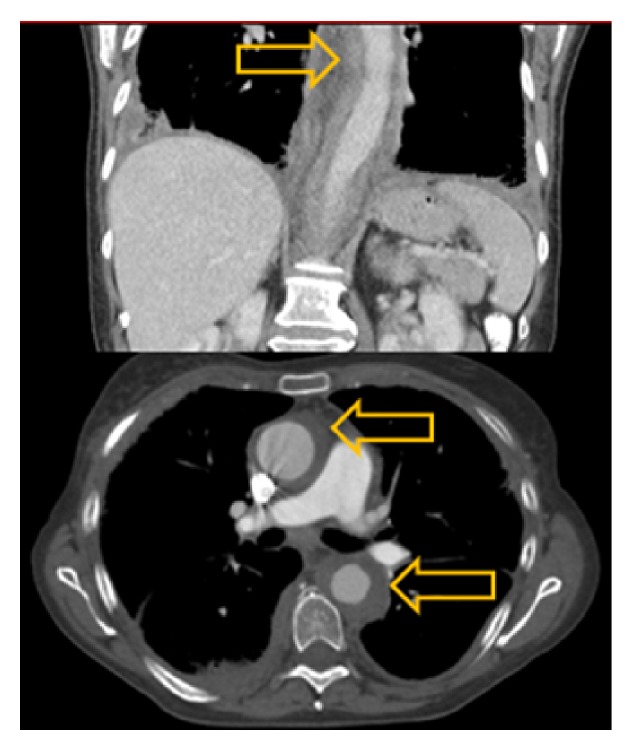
Chest CT with IV contrast showing soft tissue density surrounding the entirety of the thoracic and abdominal aorta (arrows), consistent with large-vessel vasculitis.
